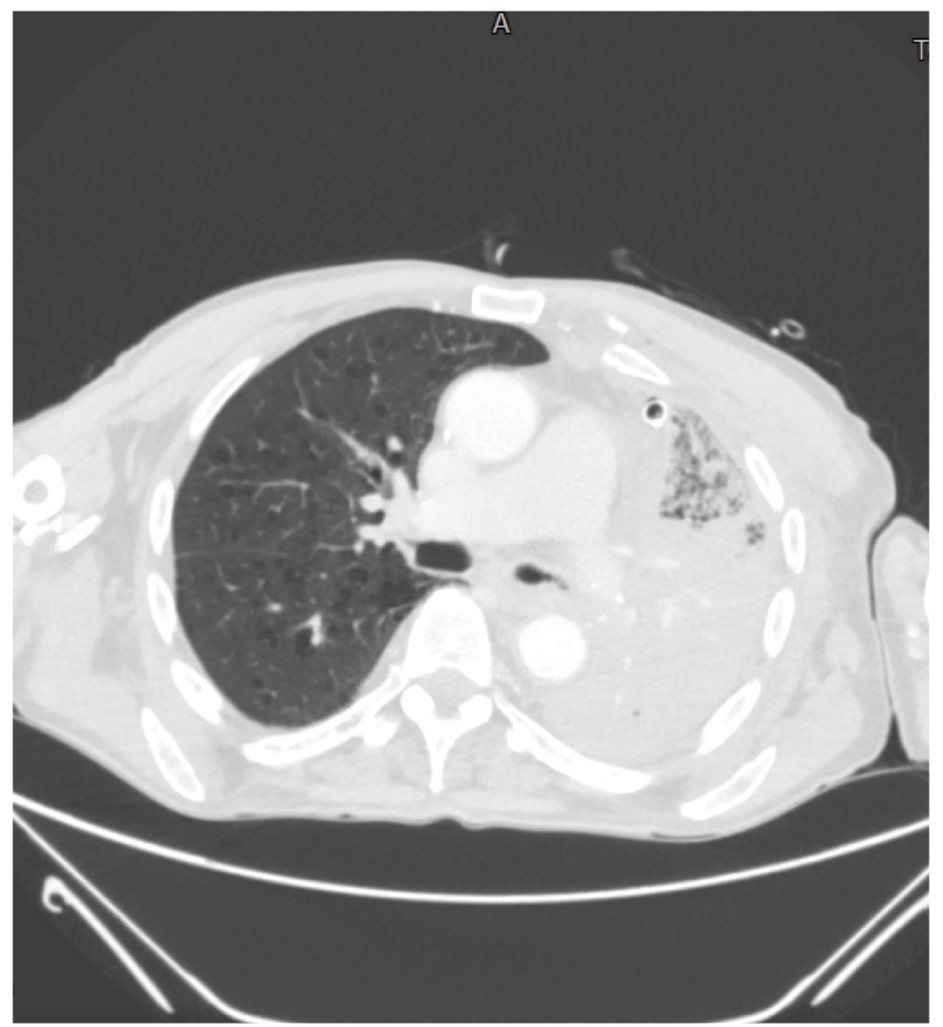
An almost complete recovery revealed by a new chest CT scan.
